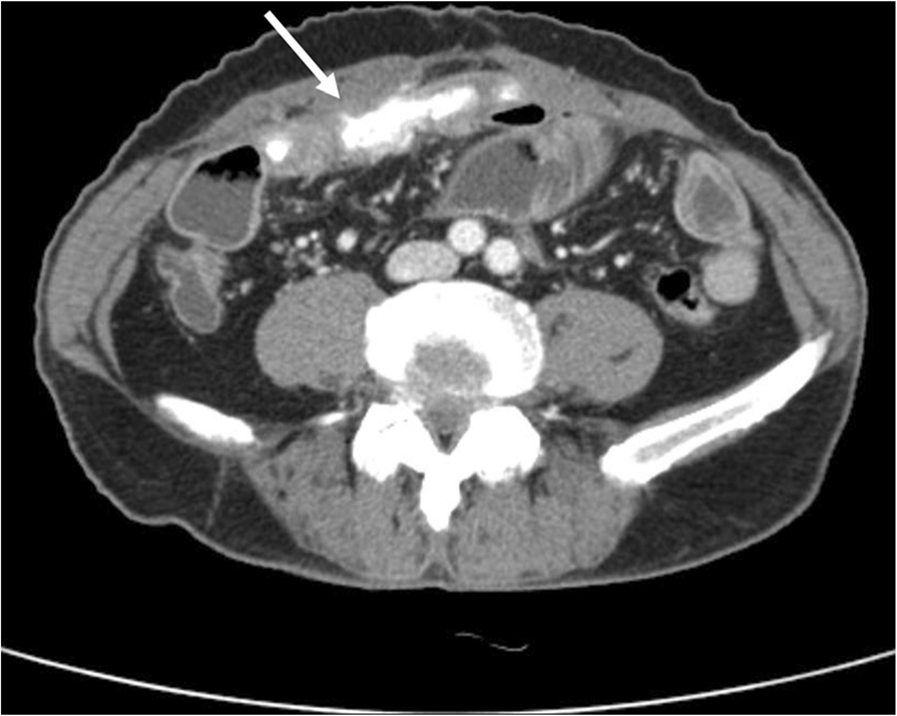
A localized high density material (arrow) in the abdominal cavity was presented in abdominal CT
